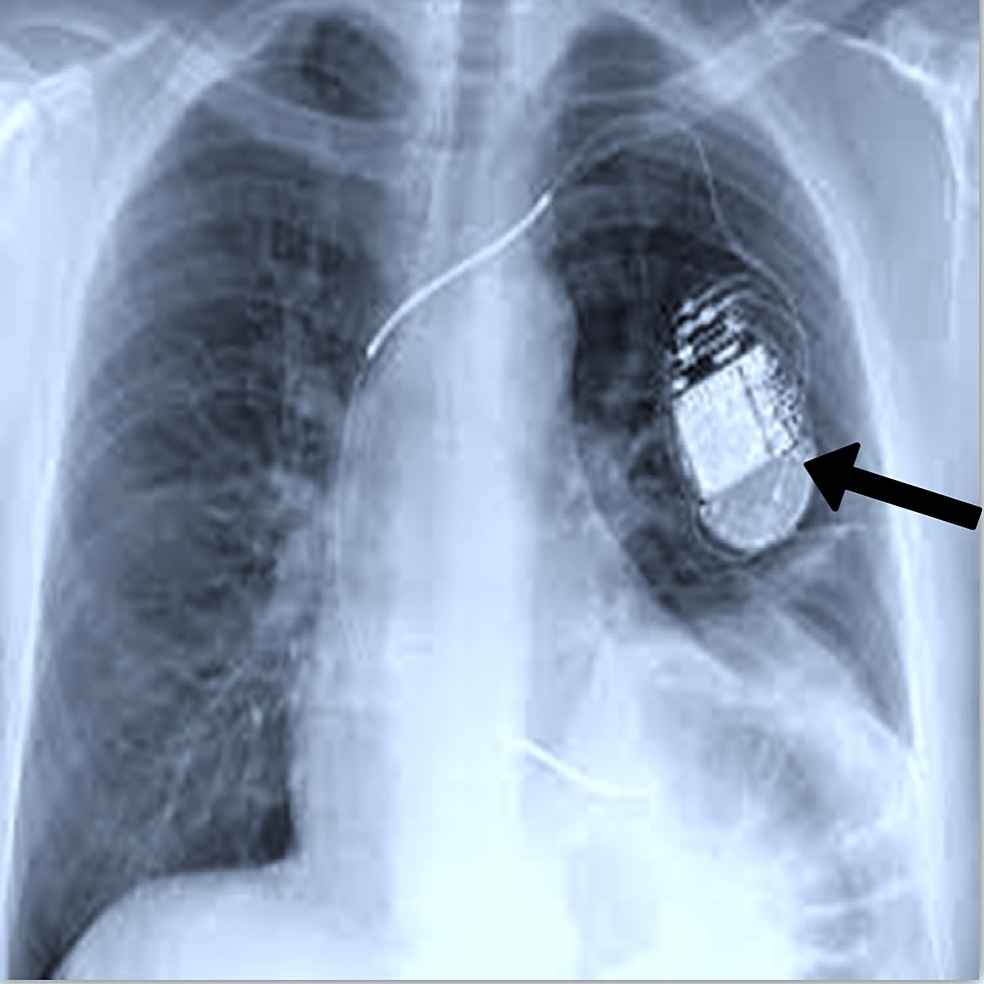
X-ray showing the pacemaker in position (arrow)
